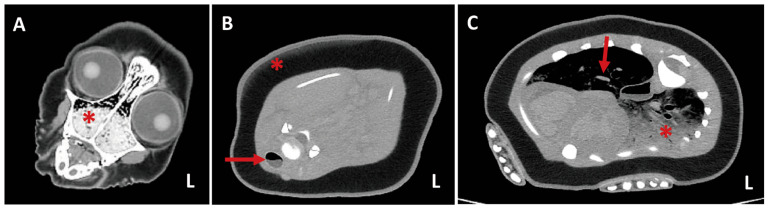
Transaxial CT findings of the cranial body of a spotted seal. (A) Head region showing frothy fluid within the nasal cavity (asterisk). (B) Section at the scapular level demonstrating fluid within the tracheal lumen (arrow) and superficial subcutaneous blubber contusion in the right periscapular region (asterisk). (C) Ground-glass opacity in the left lung (asterisk) with intrabronchial fluid accumulation (arrow). Dependent changes possibly related to livor mortis in the left lung are also noted, likely influenced by a dorsoventral position with slight leftward tilt.
